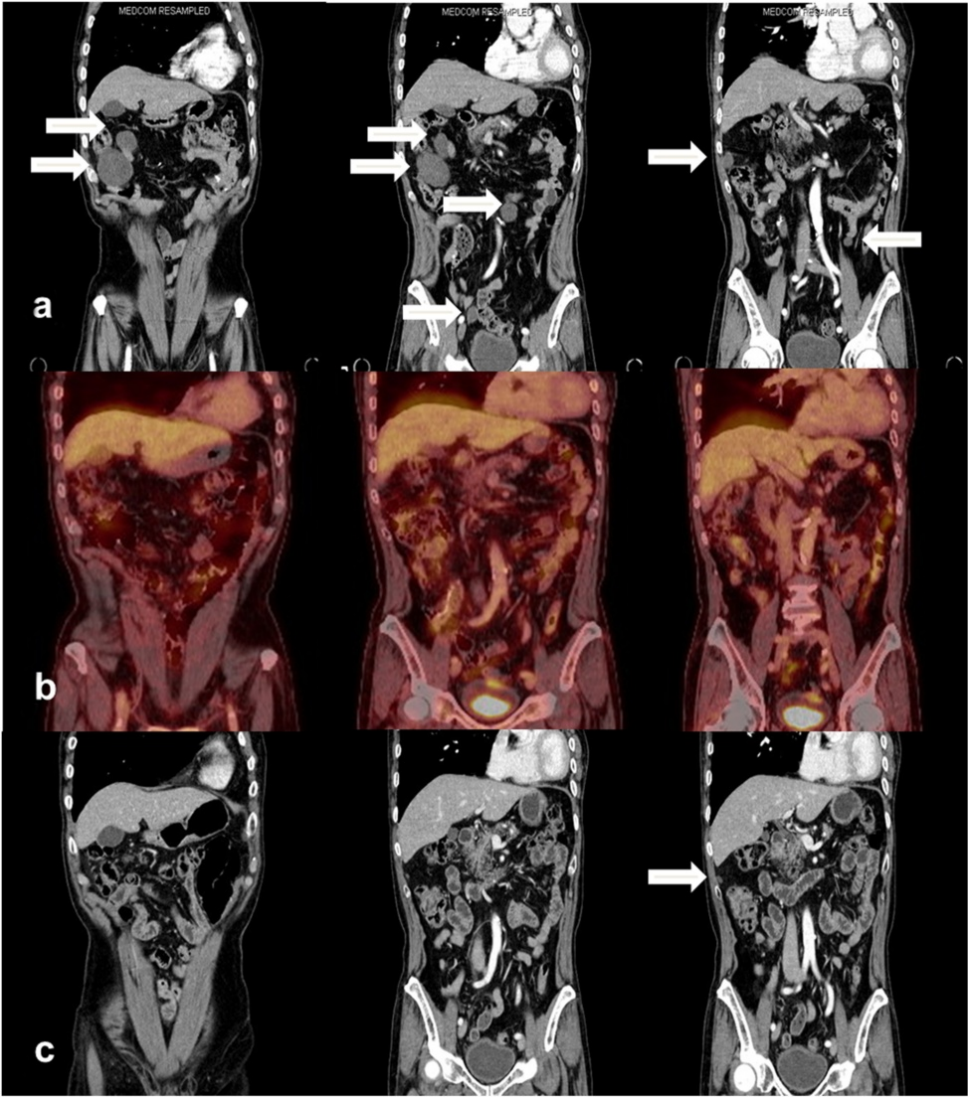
Coronal images of computed tomography or positron emission tomography scans showing a regression of peritoneal metastases. (a) Contrast-enhanced abdominal computed tomography scan showing multifocal metastatic peritoneal nodules (white arrows) in September 2013. The patient completed intraperitoneal radiotherapy followed immediately by tumor boost radiotherapy. (b) Surveillance by positron emission tomography scan, obtained 8 weeks after completion of radiotherapy, showed a complete metabolic response of metastatic peritoneal lesions. (c) A contrast-enhanced computed tomography scan, obtained in March 2014 after completion of radiotherapy, shows that most of the metastatic peritoneal lesions had disappeared.
